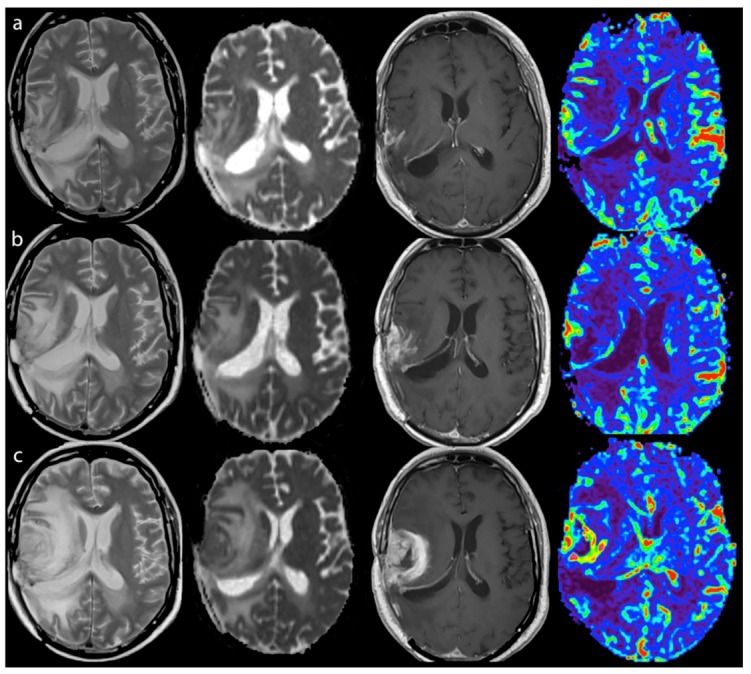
True tumor progression during immunotherapy of a LowNK and Unmethylated MGMT patient - Left to right: T2, ADC map, T1-enhanced and CBV map. (a) Oct 2013 after surgery and radio-chemotherapy and first four vaccinations, MRI-2 mo (Steroid dose 3 mg Dexamethazone, clinical condition stable): small GBM residual showing contrast enhancement (T1) and a spot of hyper-perfusion with high CBV (red to green), moderate edema and post-actinic alteration (T2 hyper-intensity) and slight ADC restriction; (b) Jan 2014, MRI-6 mo during immunotherapy (Steroid dose 3 mg Dexamethazone, clinical condition stable): enlargement of enhancing, of hyper-perfused and of ADC restricted volumes and of edema; (c) Mar 2014, MRI-8 mo after immunotherapy (Steroid dose 8 mg Dexamethazone, clinical condition worsening): further extension of enhancement with thick ring pattern due to central necrosis, more intense and wide hyper-perfusion and ADC restriction of the enhancing lesion, increased edema with mass effect. CBV, Cerebral Blood Volume; ADC, Apparent Diffusion Coefficient; MGMT, O (6)-methylguanine-DNA methyltransferase.
